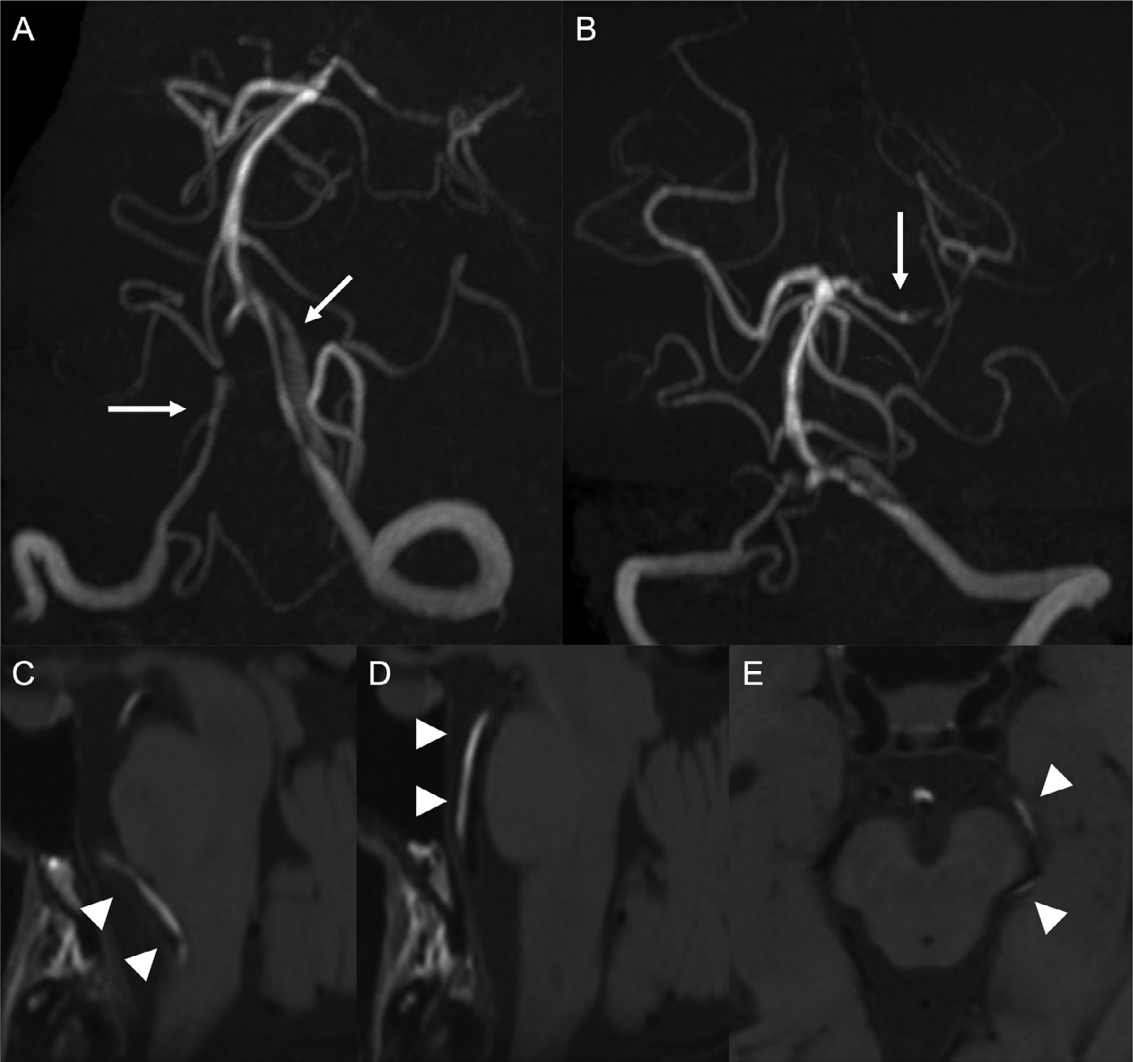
Magnetic resonance imaging on day 15 of admission showing progressive stenosis of bilateral vertebral arteries (arrows) (A) and the left posterior cerebral artery (arrow) (B). Intramural hematoma was visible as a hyperintense region (arrow heads) along the left vertebral artery (C), basilar artery (D), and left posterior cerebral artery (E) on T1-weighted imaging.
